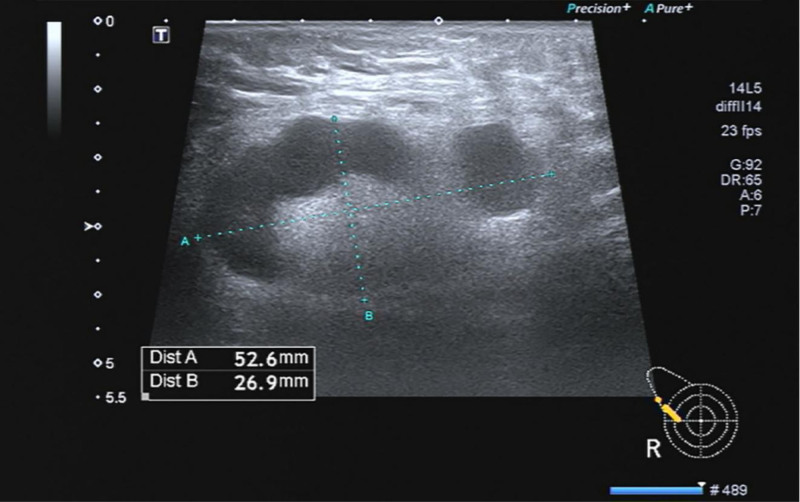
Ultrasound image showing giant lymph nodes. Superficial ultrasound revealed multiple enlarged axillary lymph nodes on both sides, and the largest lymph node was in the right axilla (size: 52.6 mm × 26.9 mm).
